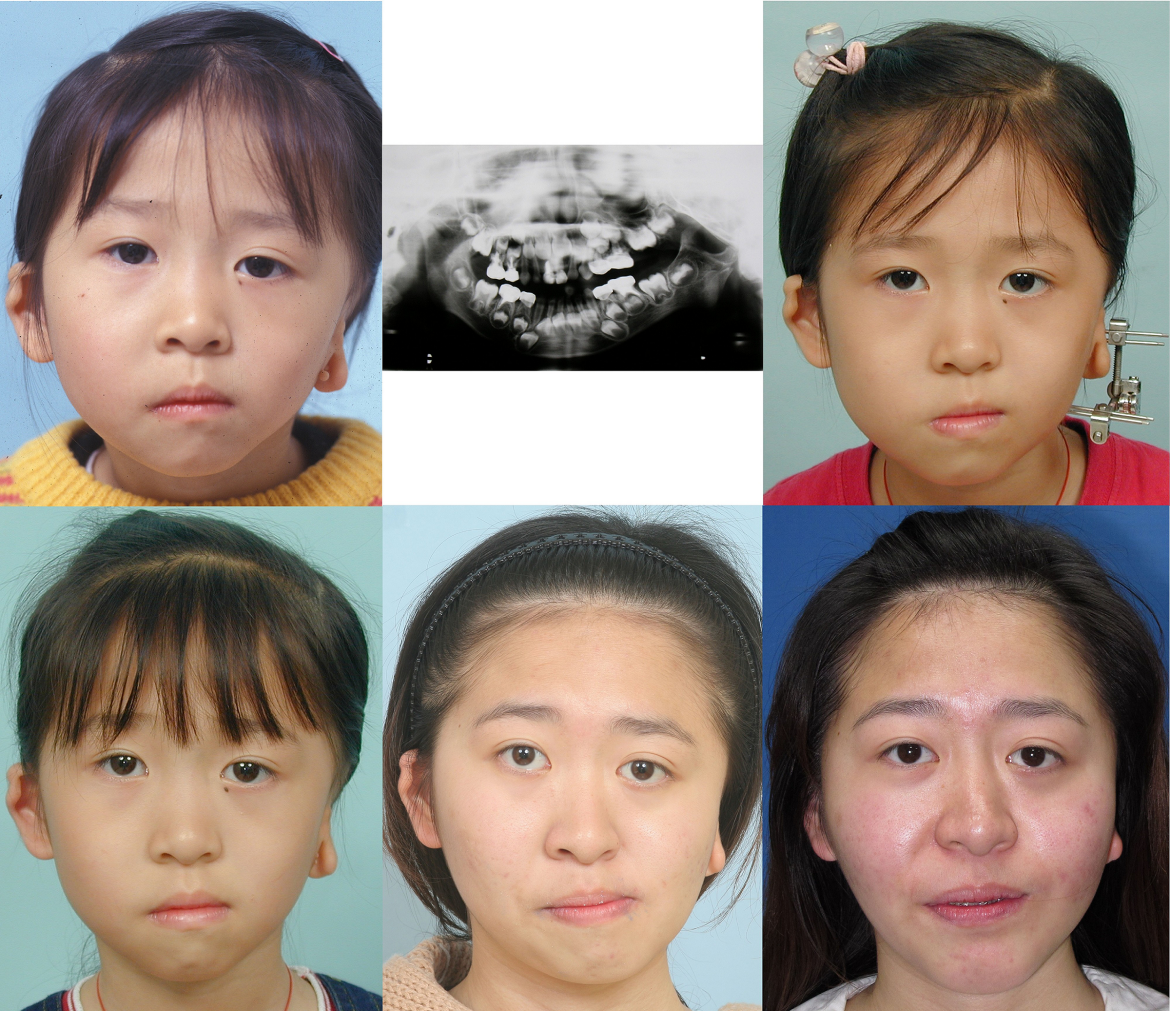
A female patient with Type IIB hemifacial microsomia involving the left side of face (case 13). She was followed up at 5 years of age (above left and middle). The panorex X-ray showed hypoplastic and inferiorly displaced condyle without adequate glenoid fossa. She was 7 years 9 months of age during the distraction osteogenesis (above right). Facial appearance was improved at 8 years 6 months of age (below left). Significant facial asymmetry was noted at 21 years 1 month of age (below middle). She received two stages of surgical correction. The facial appearance was improved at 22 years 2 months of age (below right).
